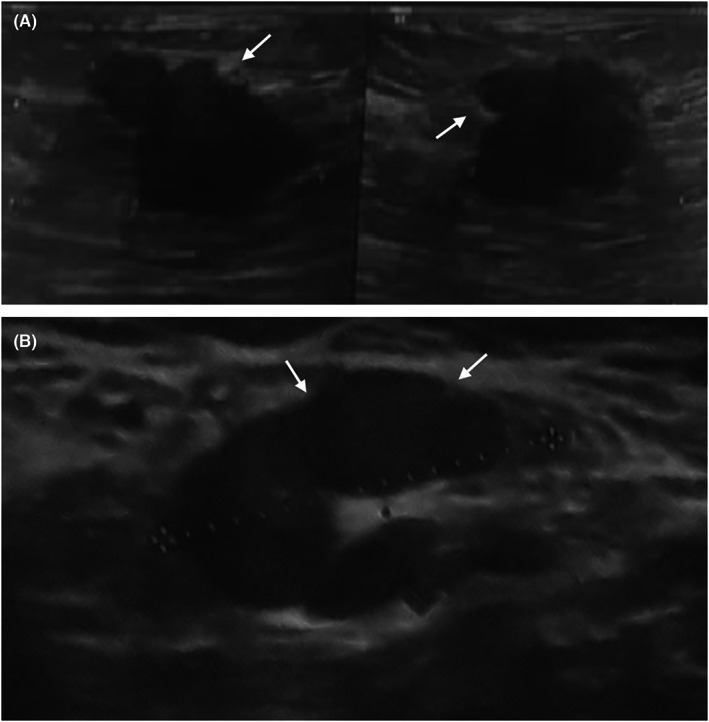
(A): A 37‐year‐old lactating woman, presented with a palpable mass with irregular shaped, microlobulated (arrowhead) margin and taller than wide orientation in ultrasound 2 months post‐partum. ER‐negative and HER2‐negative invasive ductal carcinoma was proved by Ultrasound‐guided biopsy. (B): A 37‐year‐old lactating woman with a suspicious appearing lymph node revealed a metastasis from triple‐negative invasive ductal carcinoma with ultrasound‐guided biopsy 2 months post‐partum.
